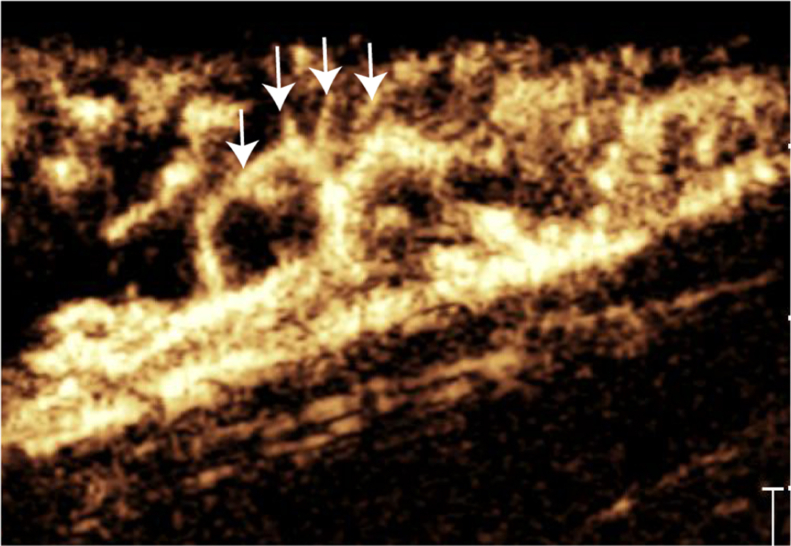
The contrast-enhanced ultrasound (CEUS) image of a lymphatic communicating branch, with the *white arrow* indicating the communicating branch.
